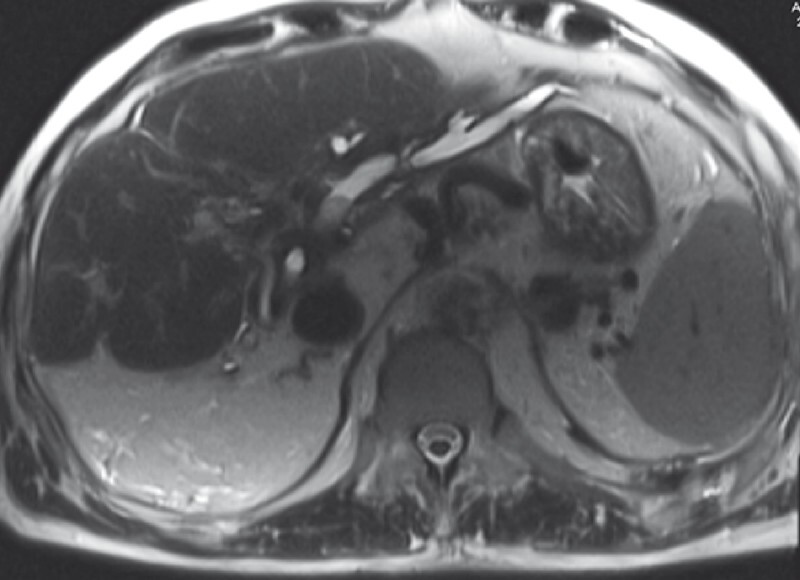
Magnetic resonance imaging showed multiple large and impacted left intrahepatic duct stones.
